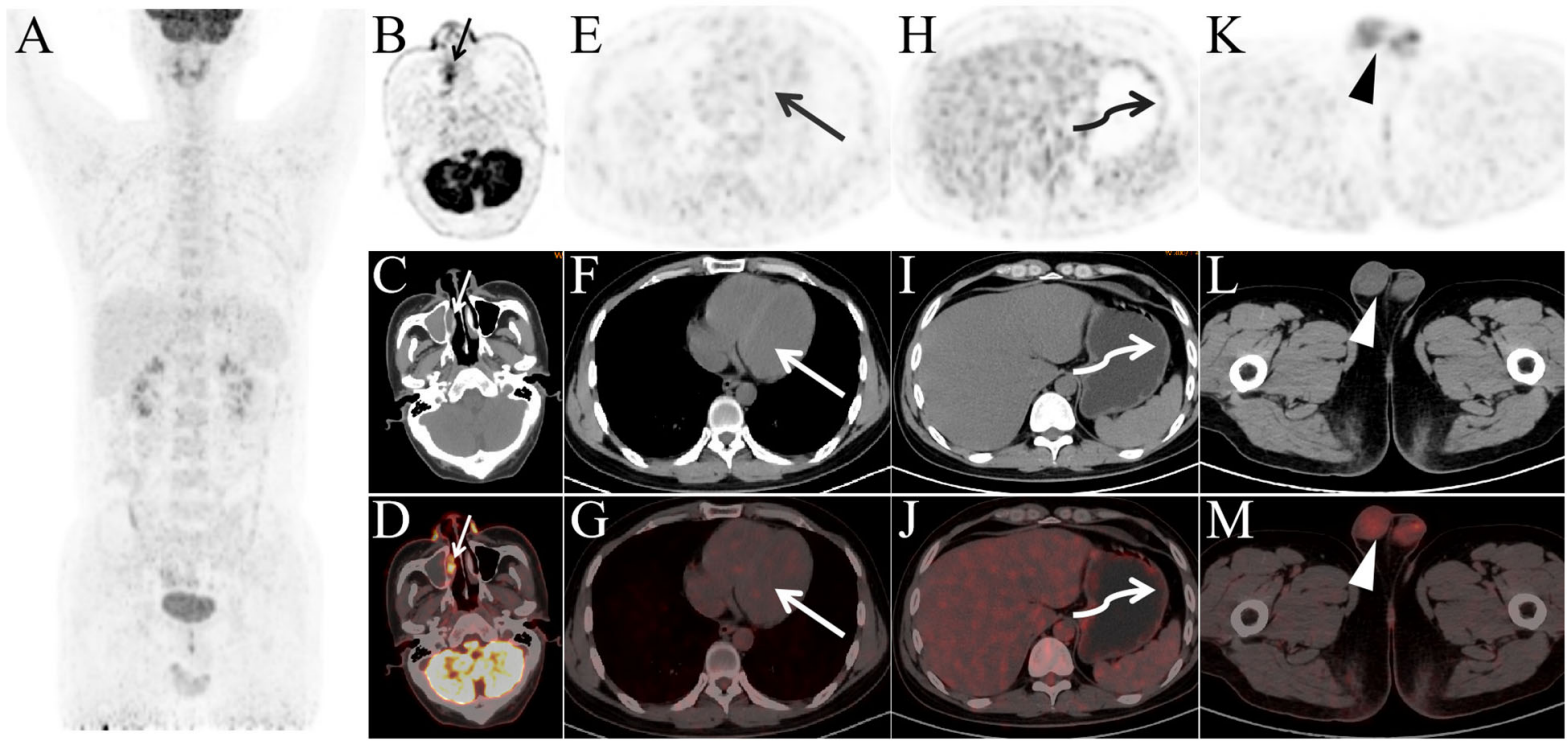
Panels (A–M) present the post-treatment PET/CT images after two cycles of therapy. Panel (A) shows that, compared to the pre-treatment images, FDG-avid lesions have nearly disappeared throughout the body. Panels (B–D) show a slight reduction in the soft tissue of the bilateral nasal cavity and right maxillary sinus, with a significant decrease in FDG uptake, SUVmax 6.3. The previously observed FDG-avid lesions in the left atrium (long arrow, SUVmax 1.0), gastric body (curved arrow, SUVmax 1.3), and right testis (arrowhead, SUVmax 2.0) no longer exhibit elevated FDG metabolism.
